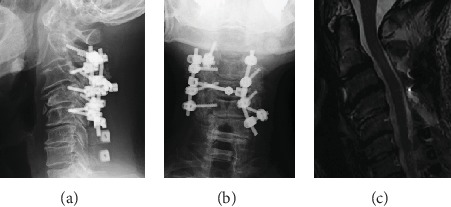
(a, b) Postoperative X-ray. X-ray shows C2-5 posterior fusion. Anterior spondylolisthesis of the axis was corrected. (c) Postoperative MRI image. MRI image shows that decompression had been performed and that the central cord deficit had been improved.
